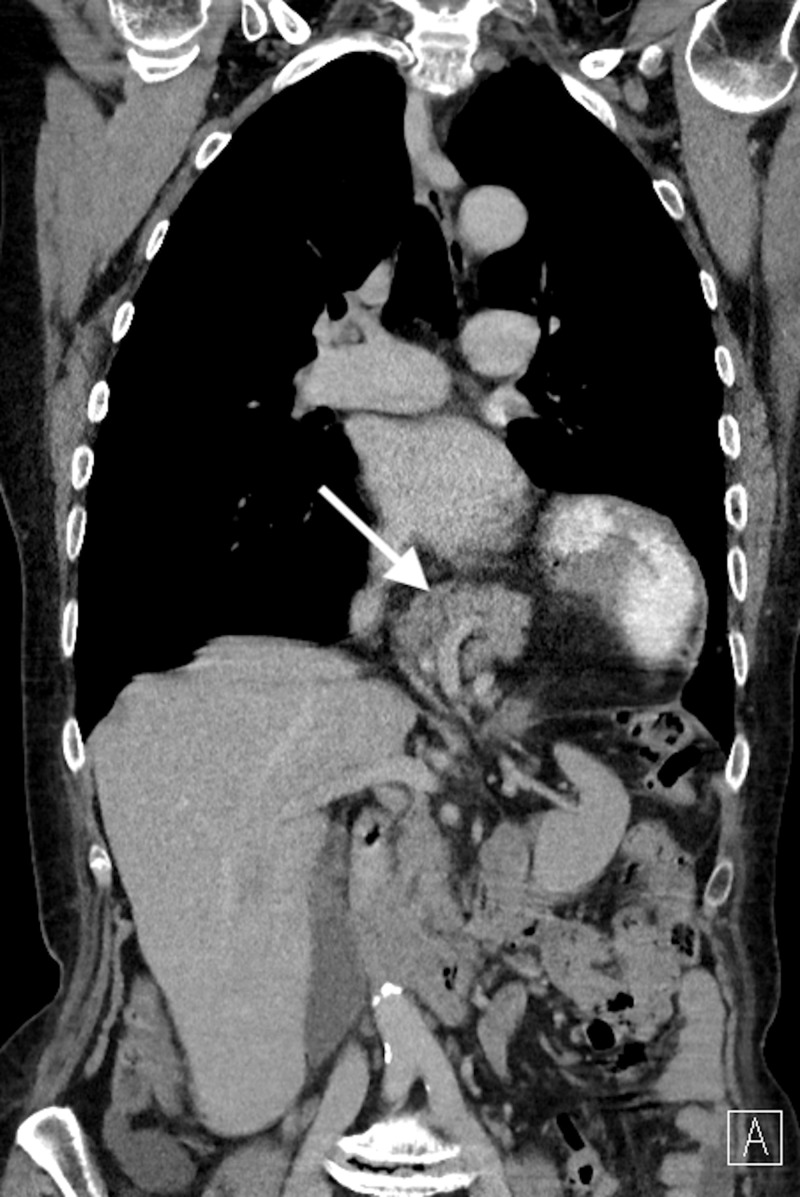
CT-scan of Patient A depicting herniation of the pancreas into the thoracic cavity.
